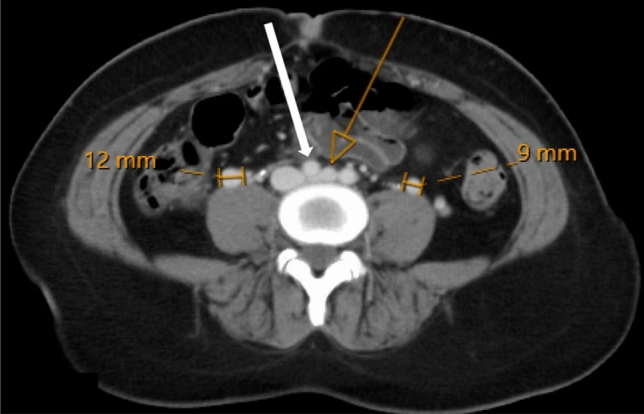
Iliac vein compression in a patient with pelvic venous disorder. 35-year-old multiparous female presenting with several months of pelvic pain and painful vulvar varicosities. Axial contrast-enhanced computed tomography shows focal narrowing of the left common iliac vein (orange arrow) by the right common iliac artery (white arrow) with associated dilated ovarian veins (left = 9 mm, right = 12 mm; orange measuring calipers)
